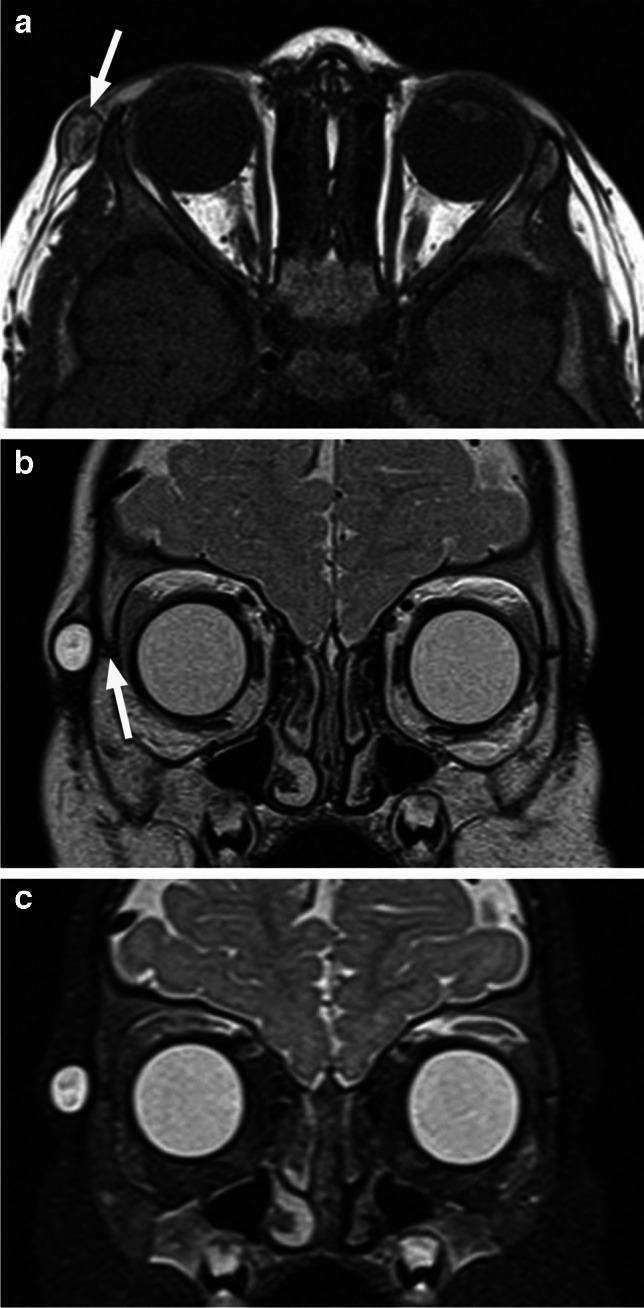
Dermoid. Axial T1-weighted image of the orbits (a) shows a well-defined mildly hyperintense oval lesion (arrow) in the typical lateral periorbital location. The mass is hyperintense on coronal T2-weighted image (b), without suppression on the corresponding STIR image (c). Arrow in (b) points to the adjacent frontozygomatic suture
